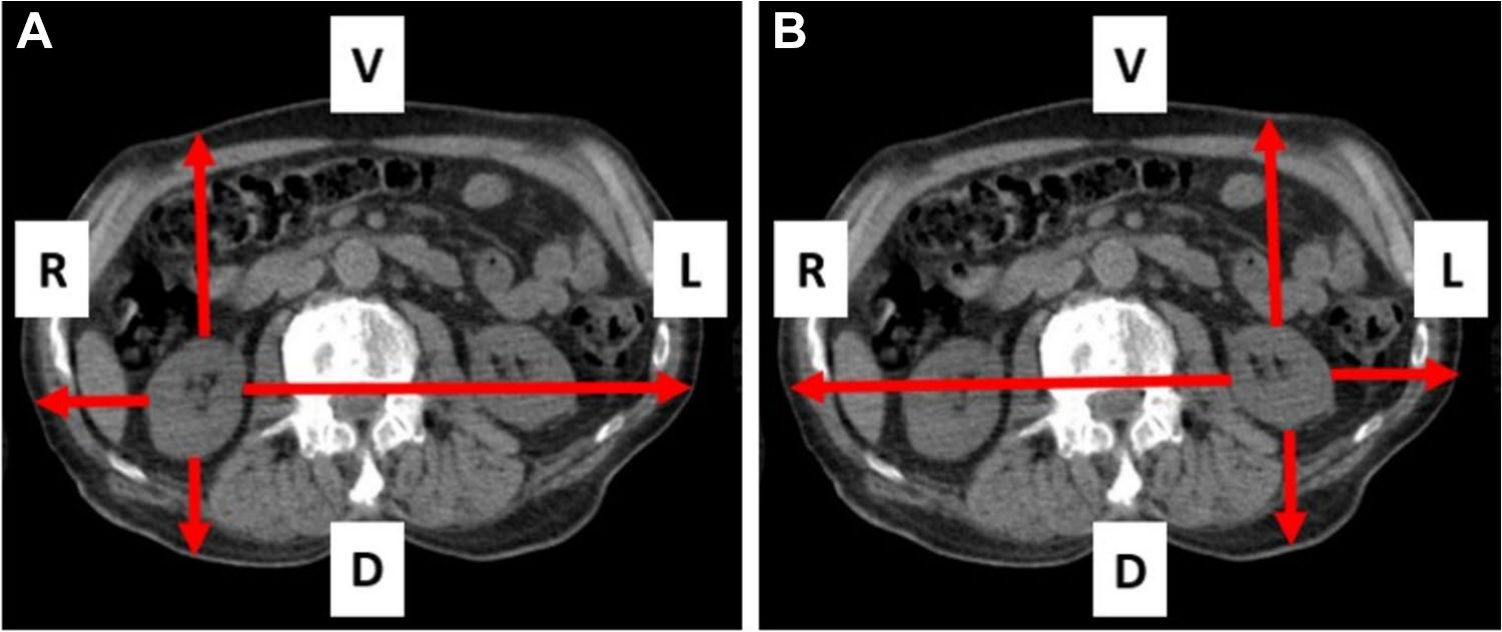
Transaxial CT-view of a 48 kg patient with measurement of the distances from the right kidney (A) and the left kidney (B) to the corresponding body surface
